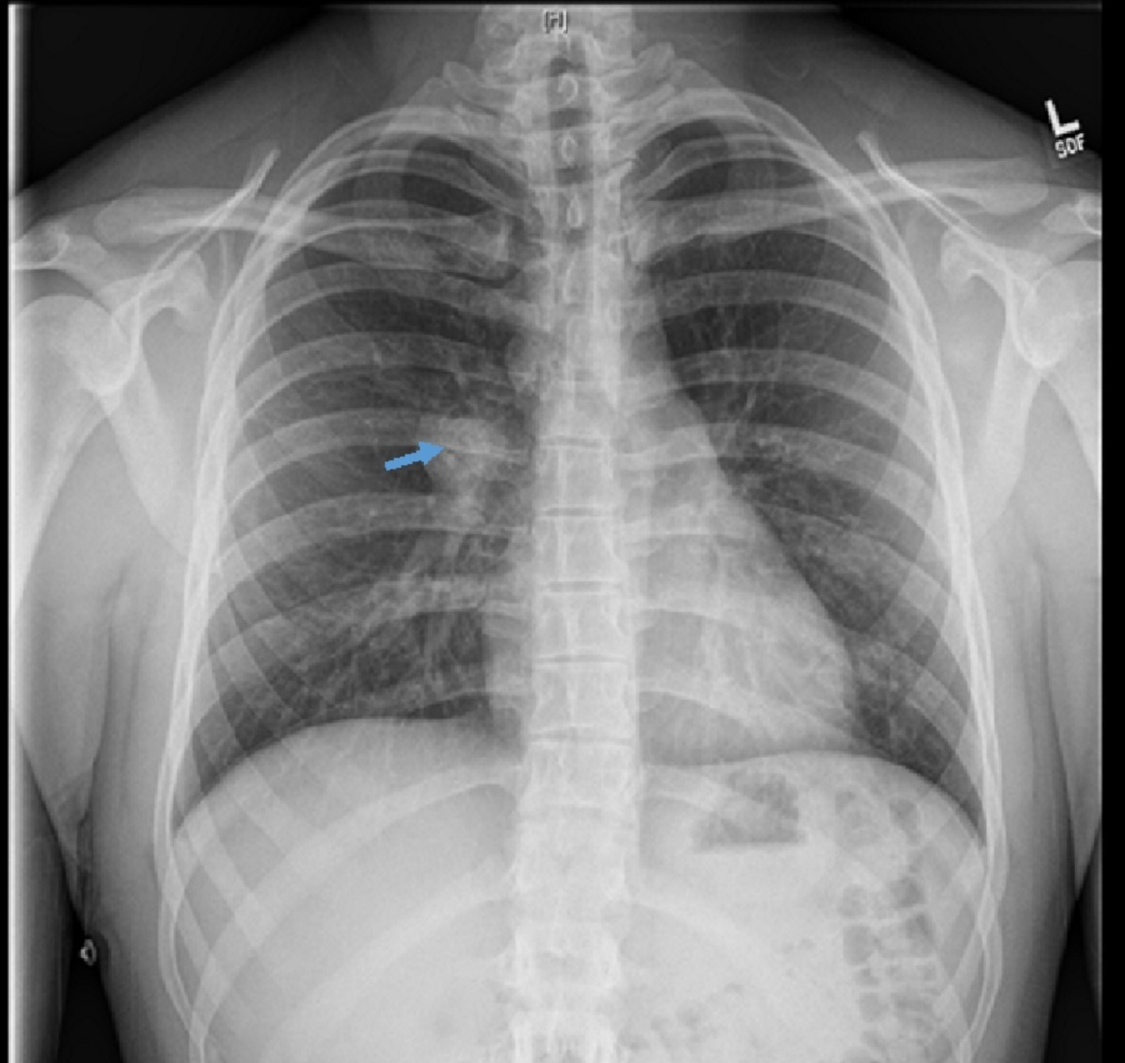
Chest X-ray showed perihilar mass (Arrow).
